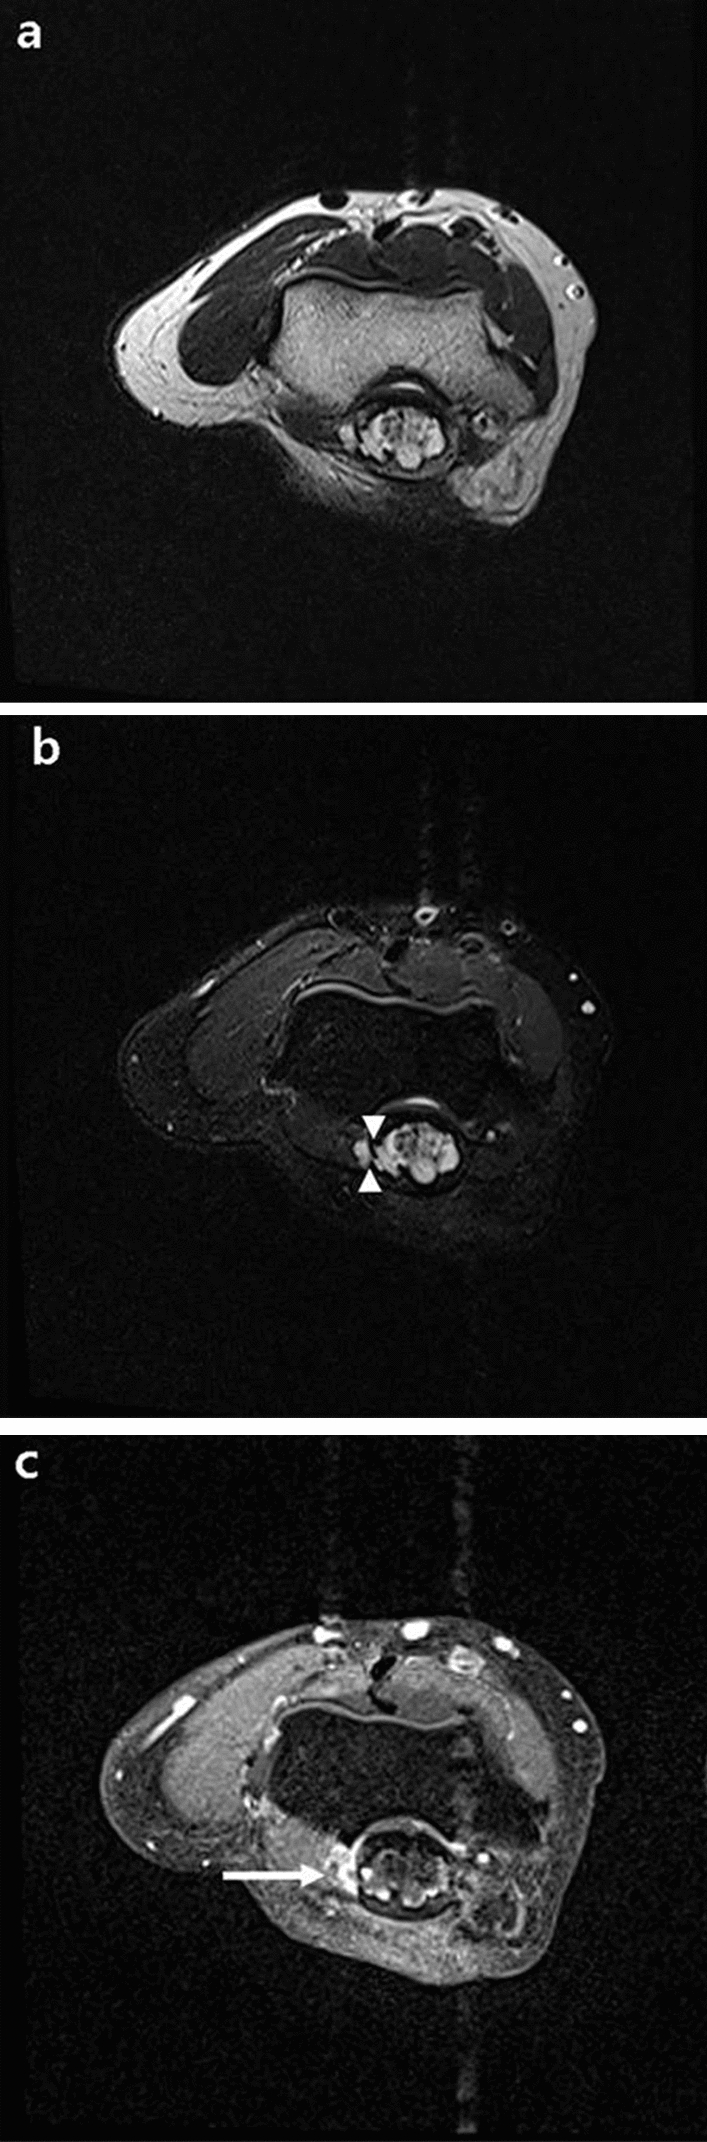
MRI of the right elbow. In-phase axial T2-weighted (T2W) (a), water only T2W (b), and T1-weighted (T1W) fat-suppressed contrast enhanced (c) images show a 1.5 × 1.3 × 2.3 cm sized intraosseous lesion with endosteal scalloping and peripheral enhancement. The lesion extended through cortical breach (white arrowheads) with enhancing extraosseous component (white arrow)
